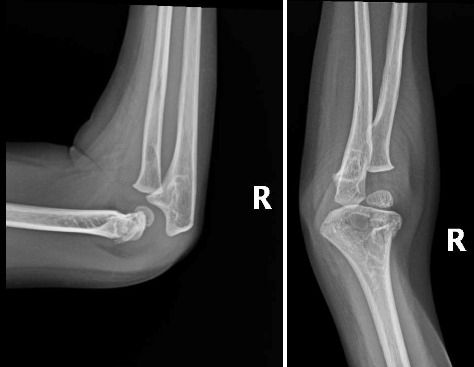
Radiographs of the right elbow.
